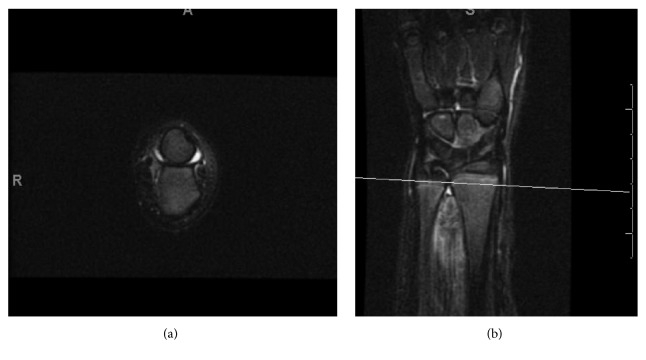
(a) Axial T2 fat suppressed and (b) coronal STIR MRI of the left wrist showing fluid in the distal radioulnar joint (DRUJ).
